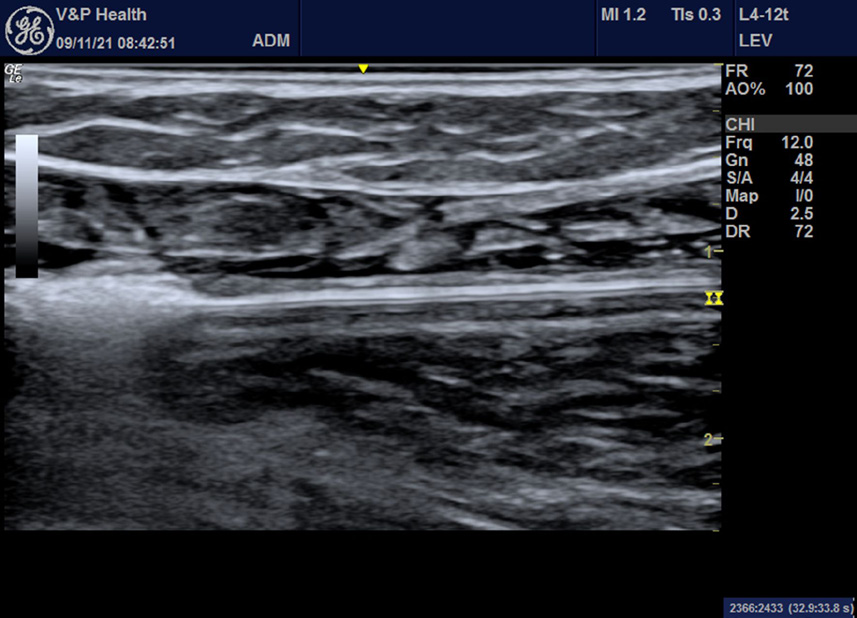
Color Doppler ultrasound image in B-mode showing the start of thermal ablation of the great saphenous vein.
